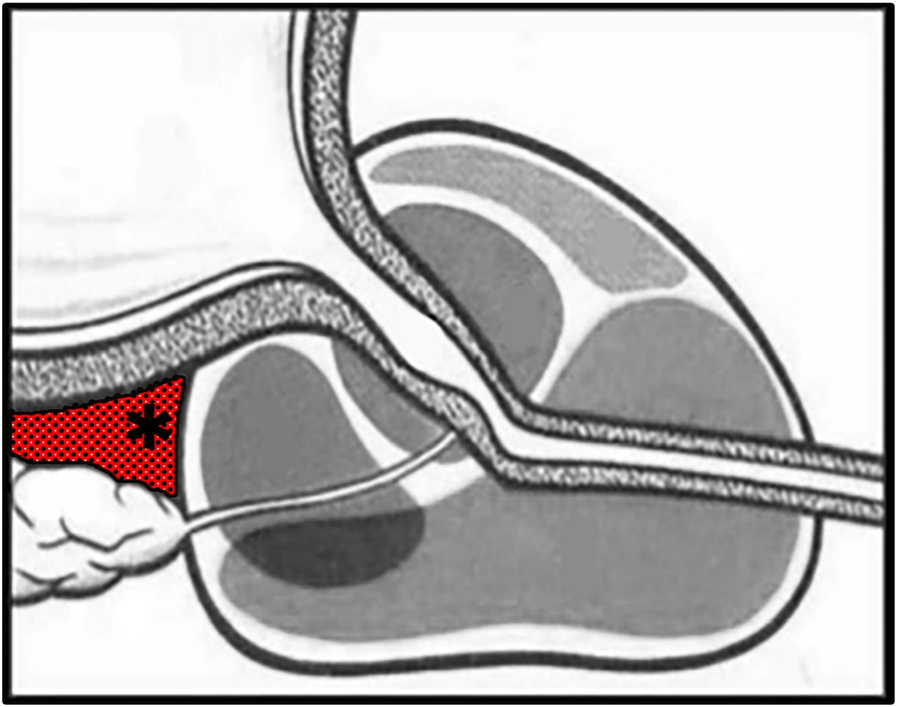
Periprostatic nerve block. Under transrectal ultrasound guidance, 2% lidocaine was injected into the triangular asterisk area where prostate neurovascular bundle passes through
